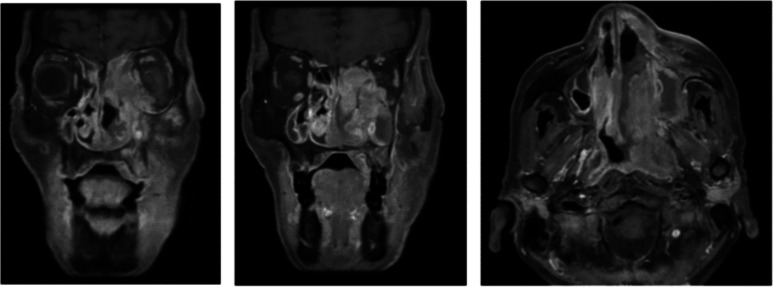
Sinus MRI. This MRI showed a large, solid mass centered in the left nasal cavity with extensive local and multicompartmental invasion, as well as subcutaneous, perineural, orbital, and intracranial spread.
